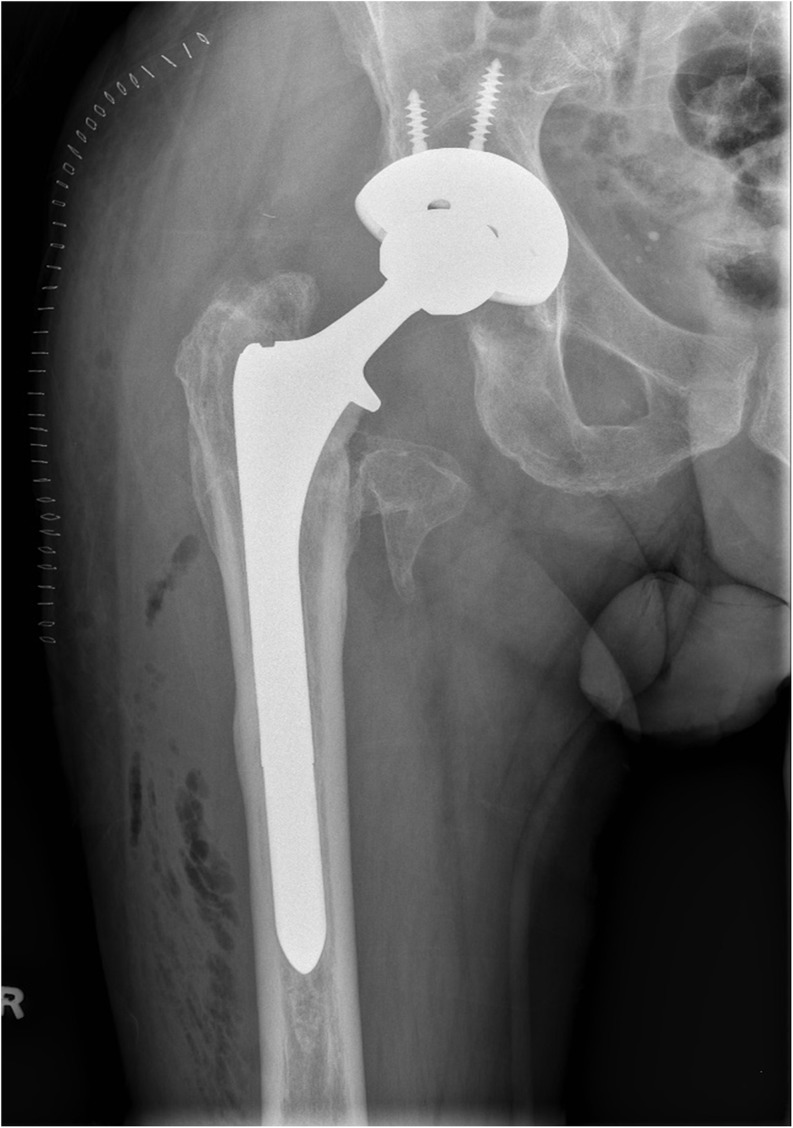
Radiographs after revision of the acetabular components.
